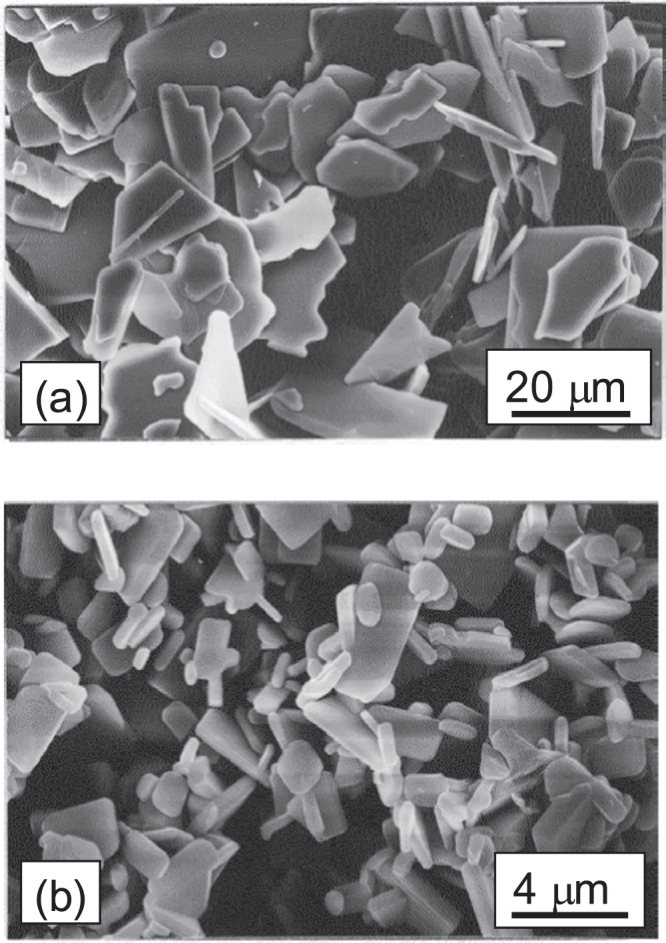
SEM images of flux-grown particles. (a) MSS–CT particles prepared at 1673 K for 8 h. (b) MSS–NT particles prepared 1573 K for 8 h.
